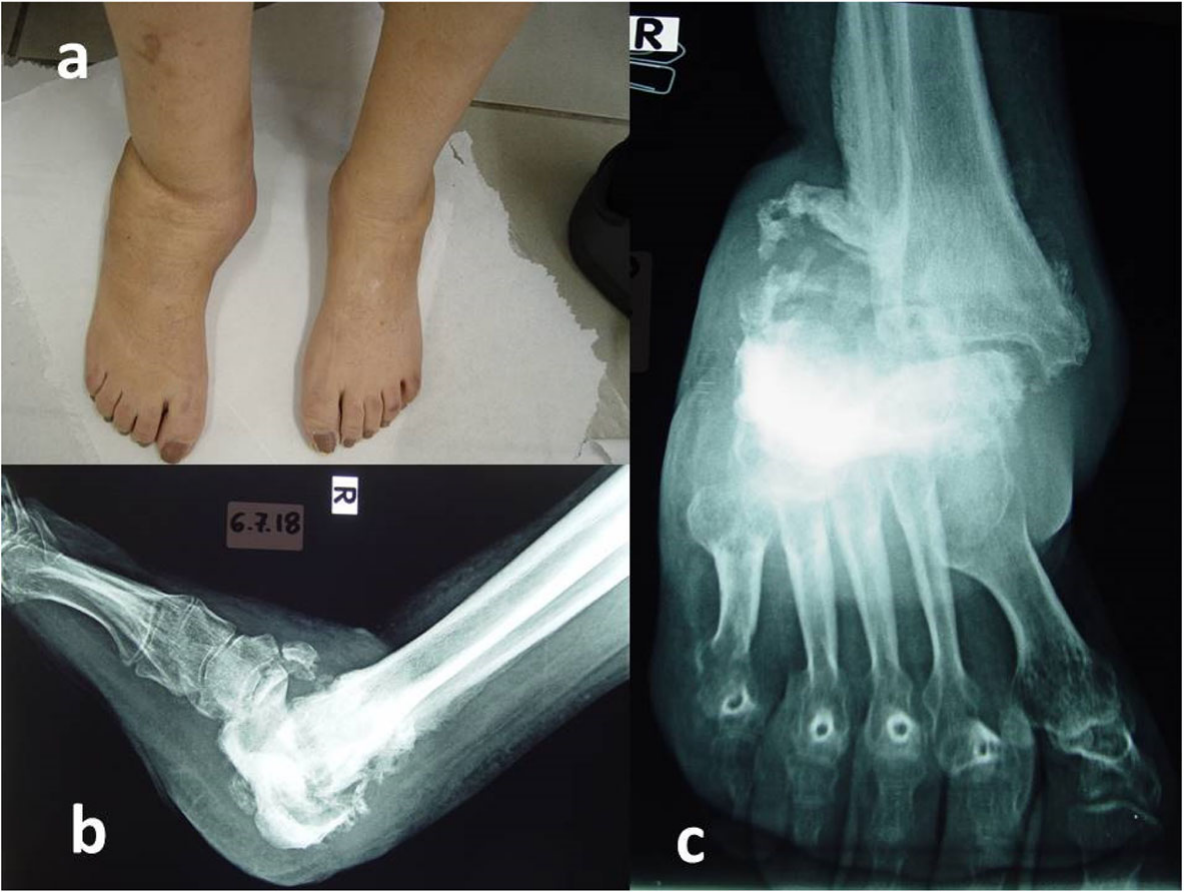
Two years later. a Excessive oedema and dislocation of right ankle. b, c X-rays: further bony destruction of the calcaneus and talus. Severe subluxation of tibial-tarsal joint. Presence of air striations of foot and lower third of extremity, possibly indicating non-aerobial infection. Further osteoporosis of the metatarsal bones
